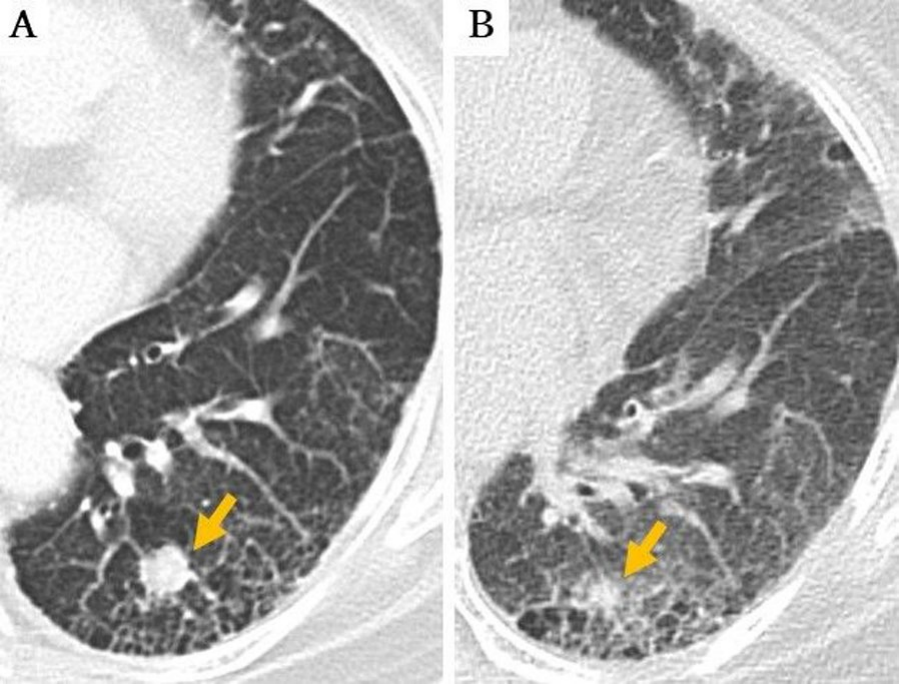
Chest computed tomography (CT) before and after CT-guided percutaneous transthoracic needle biopsy (CT-guided PTNB). A In February 2020, chest CT revealed a 1.2 × 1.2 cm solid nodule in the posterior segment of the left lower lobe. B In March 2020, follow-up CT revealed that the nodule regressed in size from 1.2 × 1.2 cm to 0.6 × 0.6 cm
